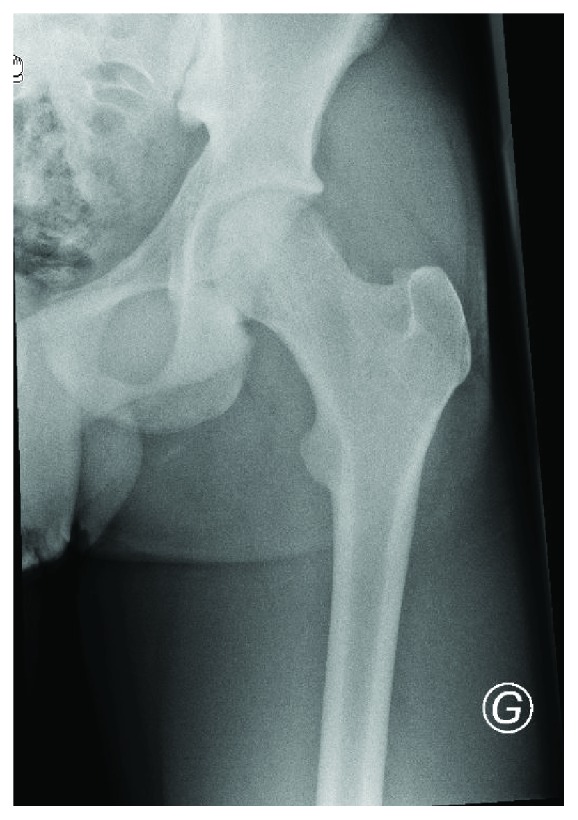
Left hip postoperative X-ray AP view.
